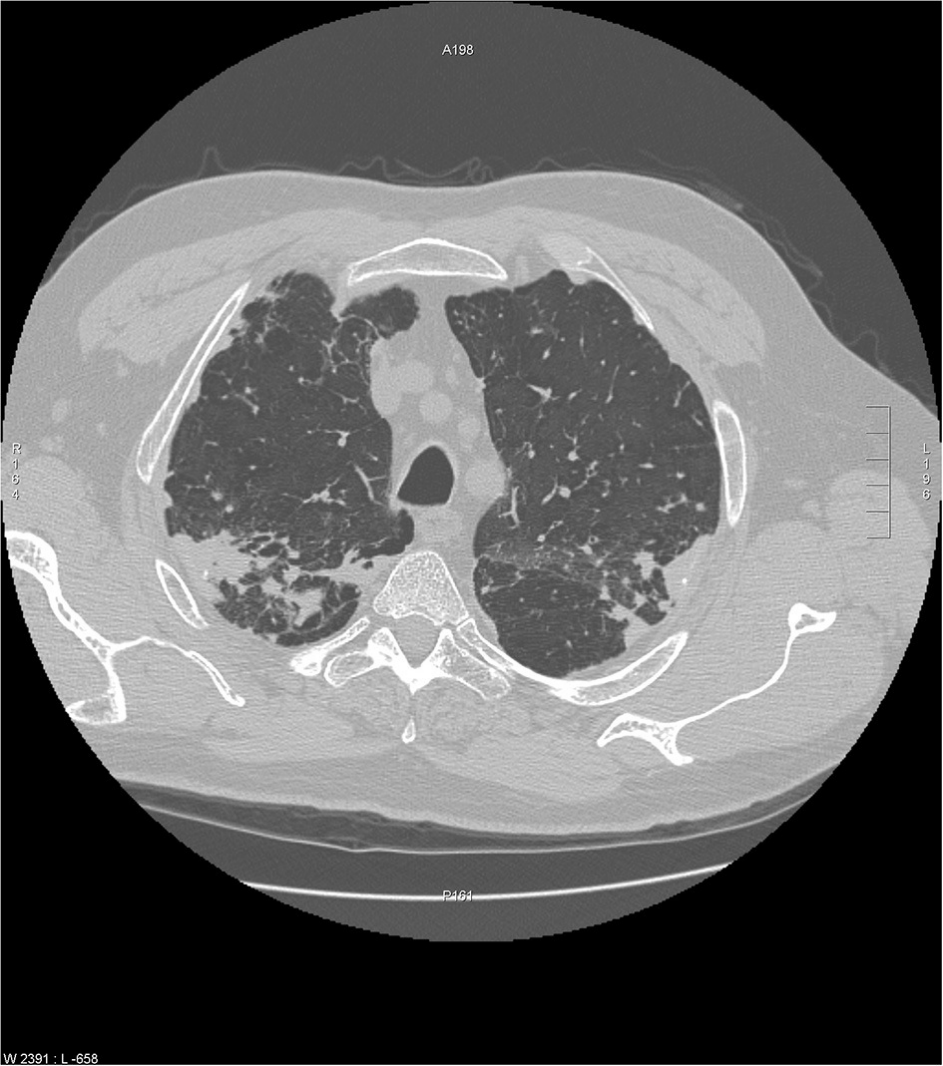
Another chest CT scan image from the same scan as Figure 6 showing peripheral and subpleural opacities. Reproduced with permission from Judson (52).
